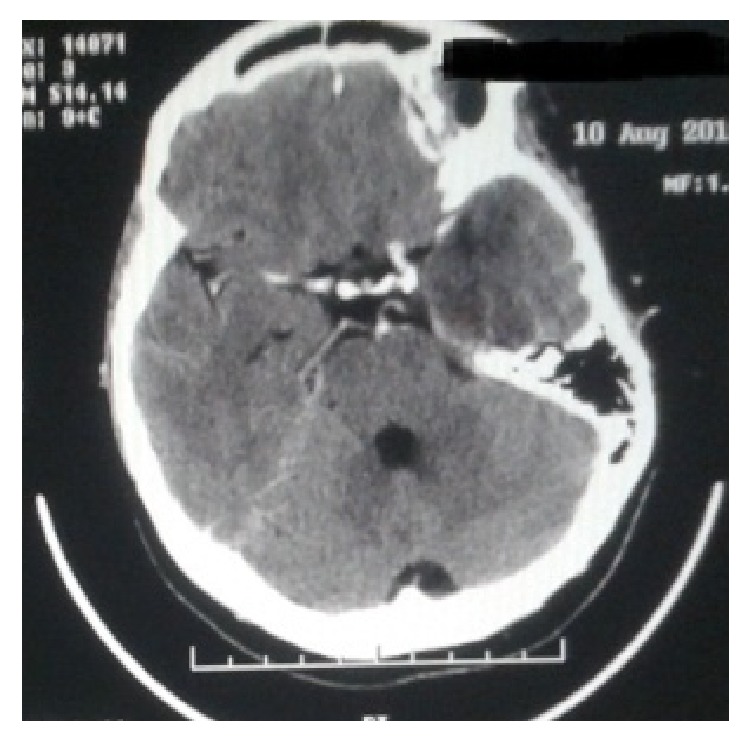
CT brain scan. Hypodense depiction of the left temporal lobe.
